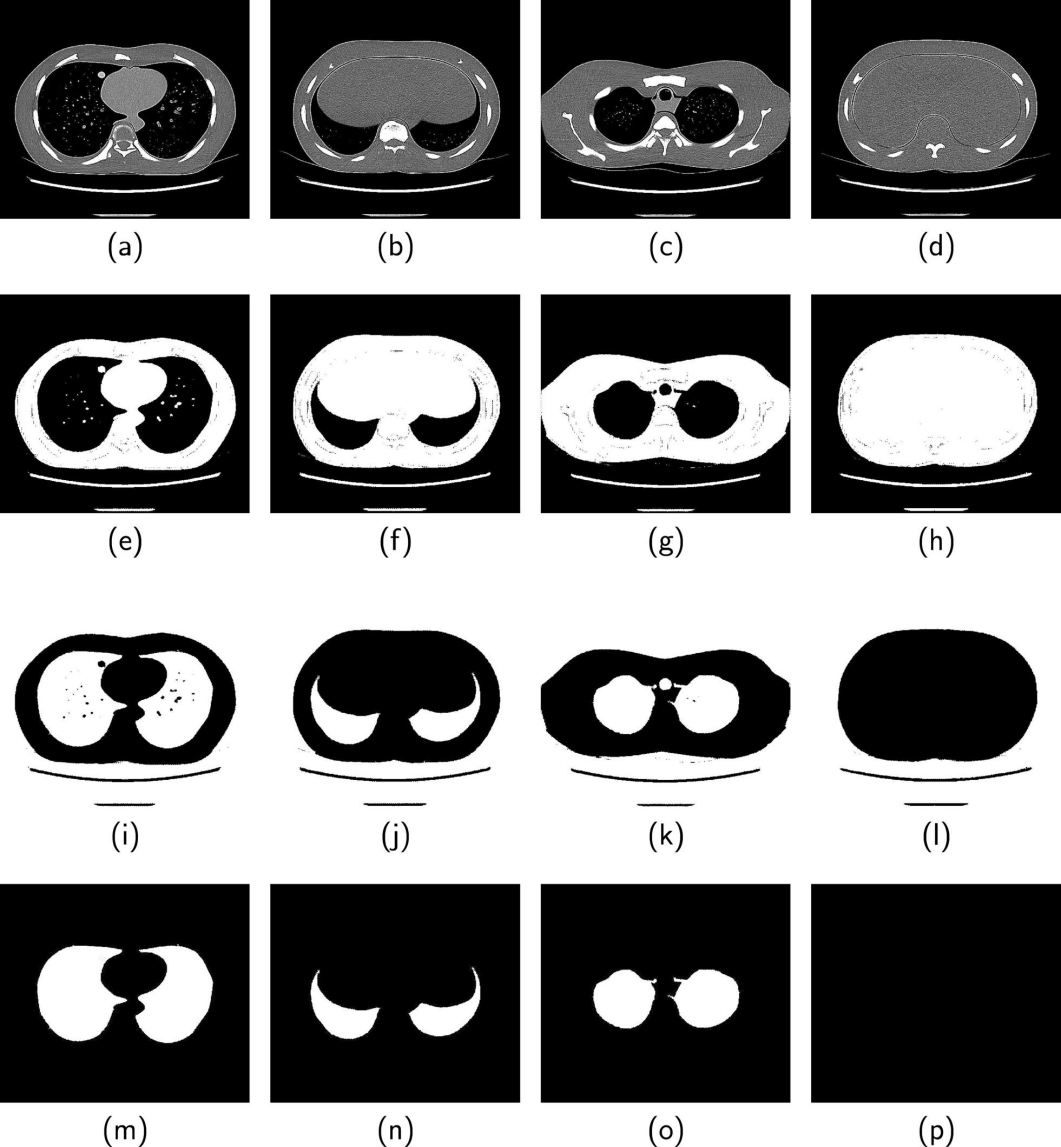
Images describing the performance of the proposed method on four CT images from the PHTM database are displayed in columns 1, 2, 3 and 4. (Row 1) Original image, (Row 2) Binarized image, (Row 3) Candidate lung region, (Row 4) Segmented lung region
